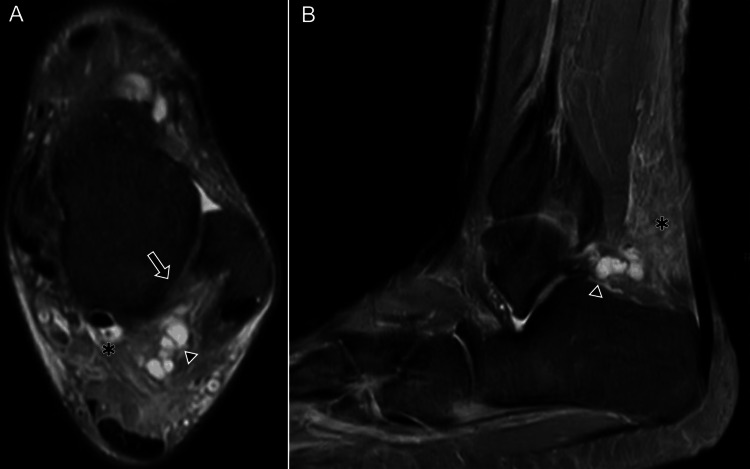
Posterior talofibular ligament (PTFL) injury with organized ganglion. A 43-year-old male: (A) axial DPFS MRI shows a thickened PTFL (arrow), a multiseptated ganglion (arrowhead), and fluid within the FHL tendon sheath (*); (B) sagittal DPFS MRI shows the organized ganglion (arrowhead) adjacent to the PTFL and heterogeneous edema of Kager’s fat pad (*). DPFS: dual proton fat-saturated sequence; FHL: flexor hallucis longus Images belong to the authors; patient consent was obtained.
